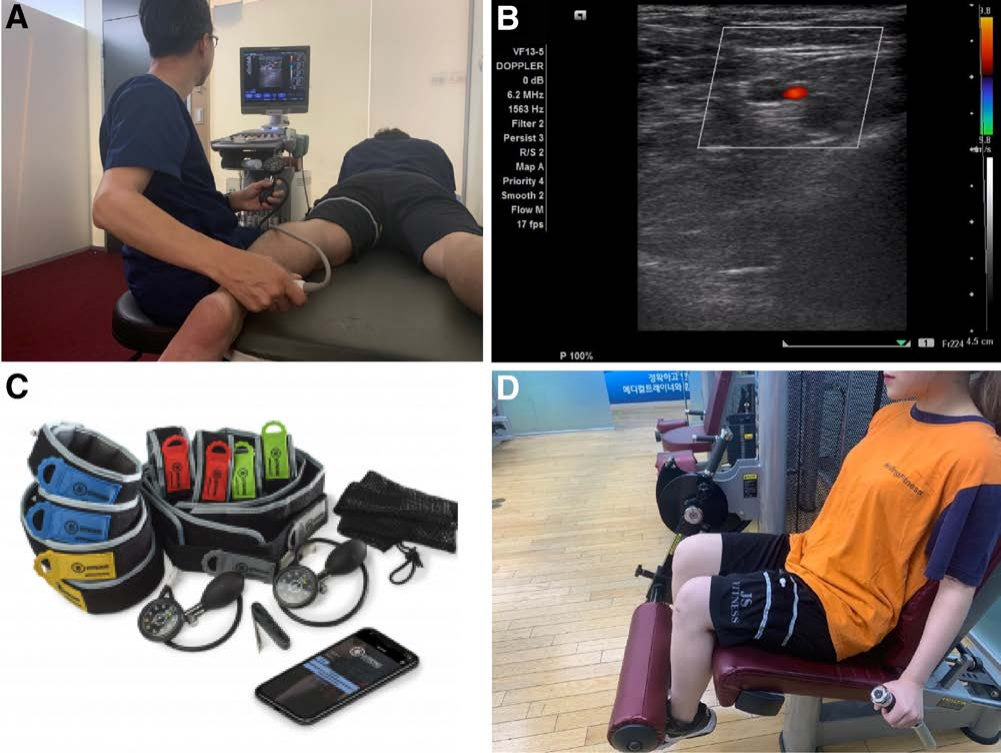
Application of the blood flow restriction. (A) Measurement of arterial occlusive pressure using Doppler ultrasound. (B) Pulsation of the posterior tibial artery. (C) Strong training system^TM^ for blood flow restriction. (D) Applying a tourniquet to restrict blood flow to the proximal femur.
